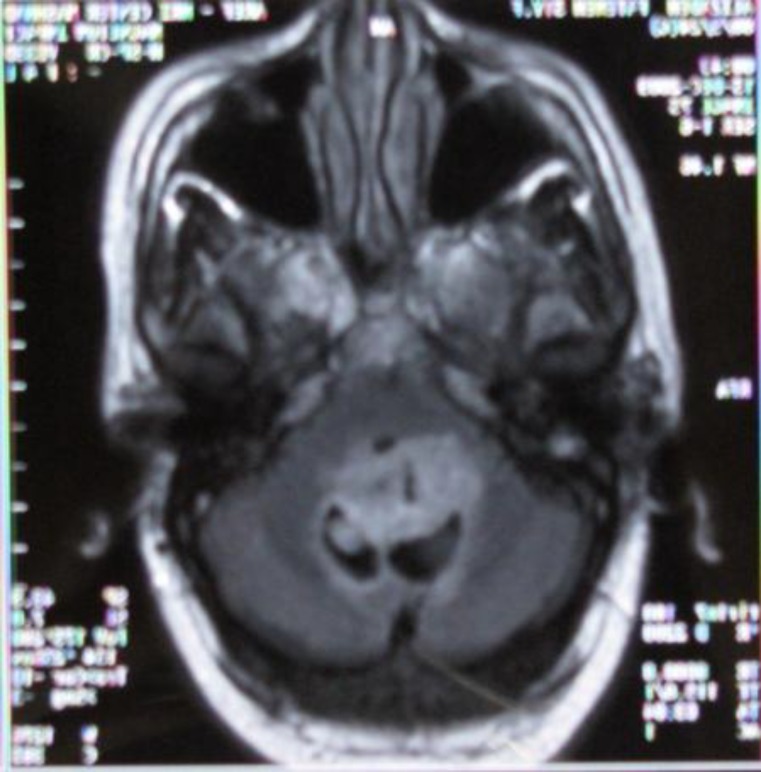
axial FLAIR MRI of the patient shows slightly hyperintense mass lesion in 4^th^ Ventricle).
